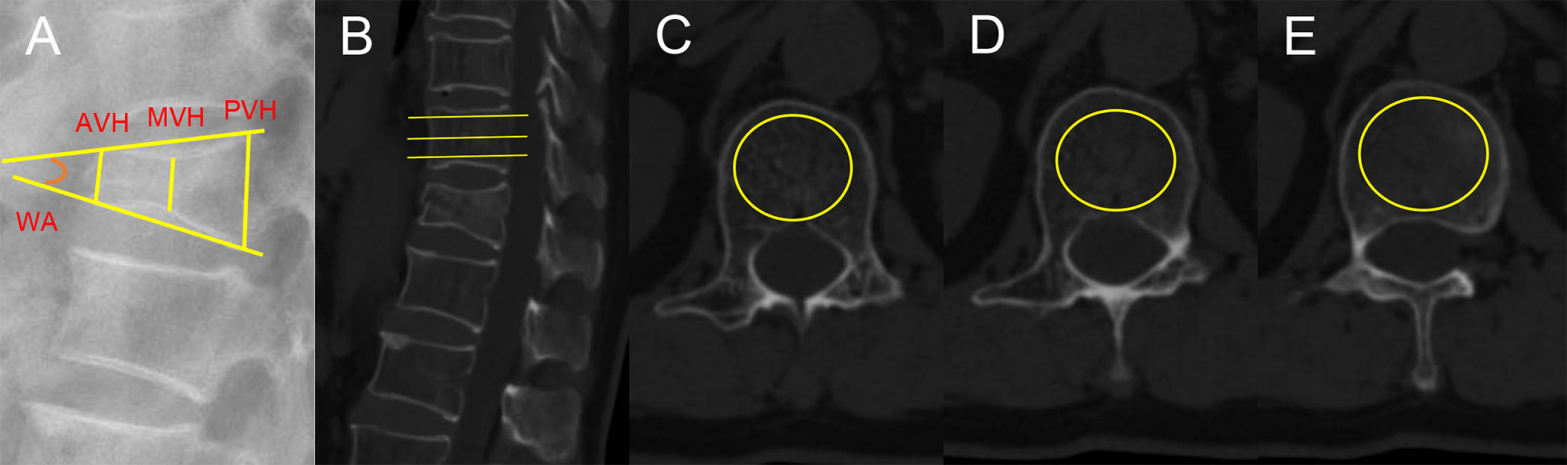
The measurement of radiographic data. (A) The AVH, MVH, PVH and wedge angle (WA) were measured on lateral X-ray. (B-E) HU value was measured on CT scanning.
